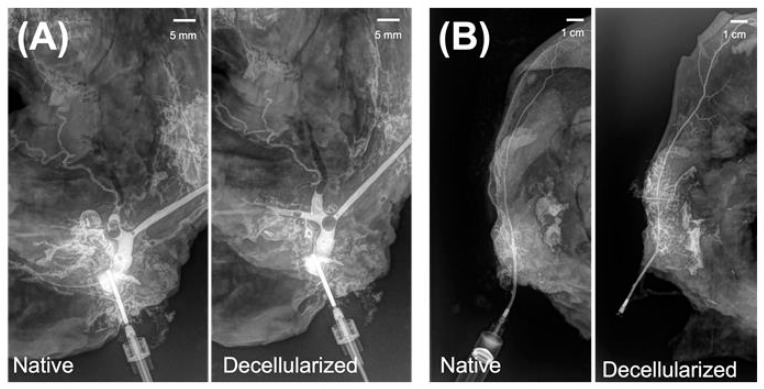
X-ray contrast angiogram of vascular network in native and decellularized face grafts. (A) Left facial artery. (B) Right temporal artery.
